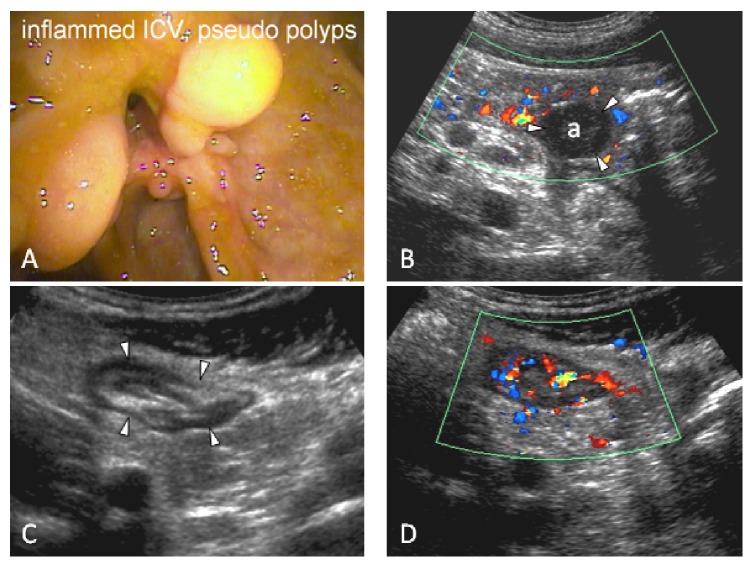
No prior diagnosis of Crohn's disease. 17-year-old male patient with no prior diagnosis of Crohn's disease. Colonoscopy is incomplete with a failure to intubate the inflamed ileocecal valve, shown in (A), with pseudopolyps. (B) Transabdominal ultrasound shows an interloop abscess cavity (a). (C) shows a thickened terminal ileum in axial view. The length of involvement (not shown) is 20 cm. (D) shows hyperaemia on colour Doppler. Interpretation: acute inflammation of the terminal ileum with a small interloop abscess. Four days after the ultrasound, the patient was admitted to hospital for intravenous antibiotic therapy.
